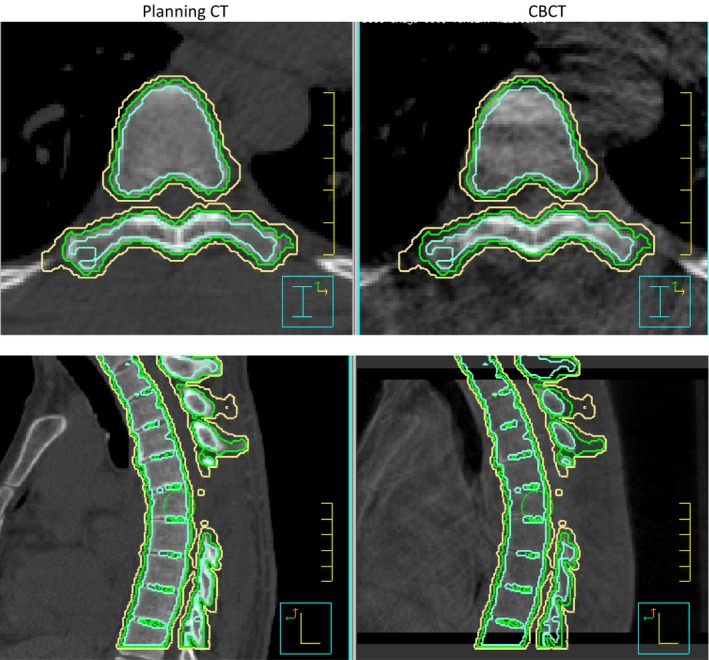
Residual error analysis for spinal column (T3‐9) of case # 16. Planning CT is on the left side and CBCT is on the right side. Green is the auto‐contour of vertebrae. Cyan and yellow are 1 mm contraction and expansion of vertebrae in planning CT. The axial and sagittal slice demonstrates the residual error is less than 1 mm for vertebrae T3‐9.
